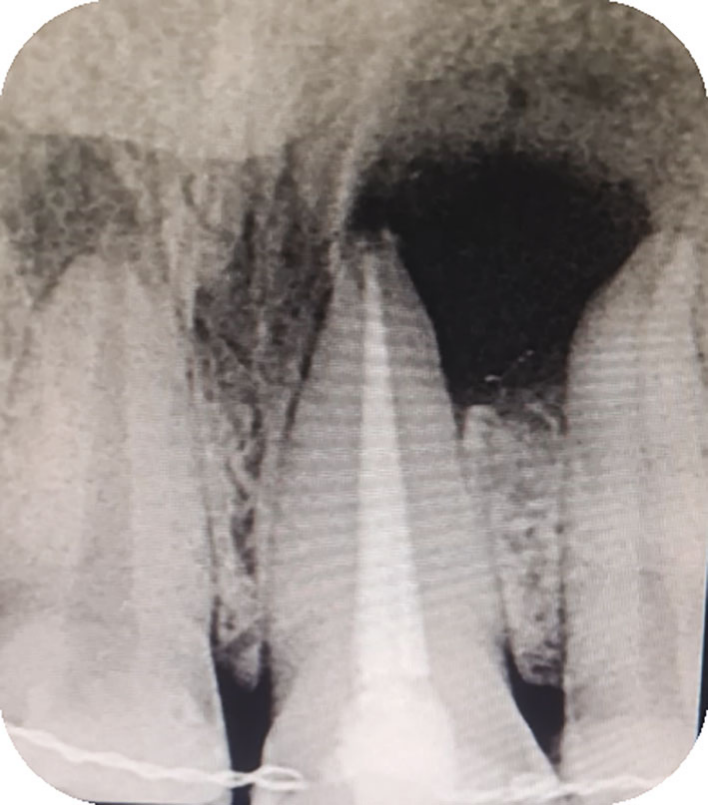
Radiographic examination showed correct root canal filling of Tooth 21.
